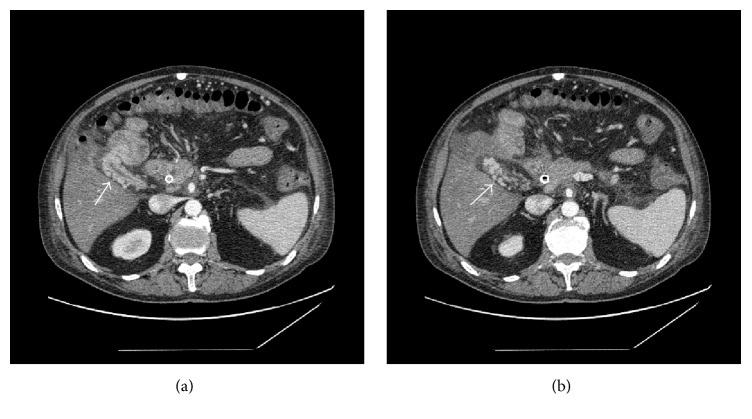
Abdominal contrast-enhanced CT, axial plane, showing gallbladder varices (diffuse variceal dilation of veins in the gallbladder wall).
